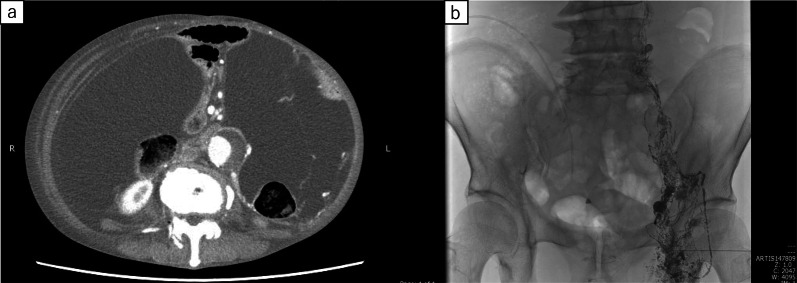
a Abdominal CT showed a large volume of ascites and no sign of cancer recurrence. b Fluoroscopic image showing injection of Lipiodol through the left inguinal lymph nodes (arrow)
